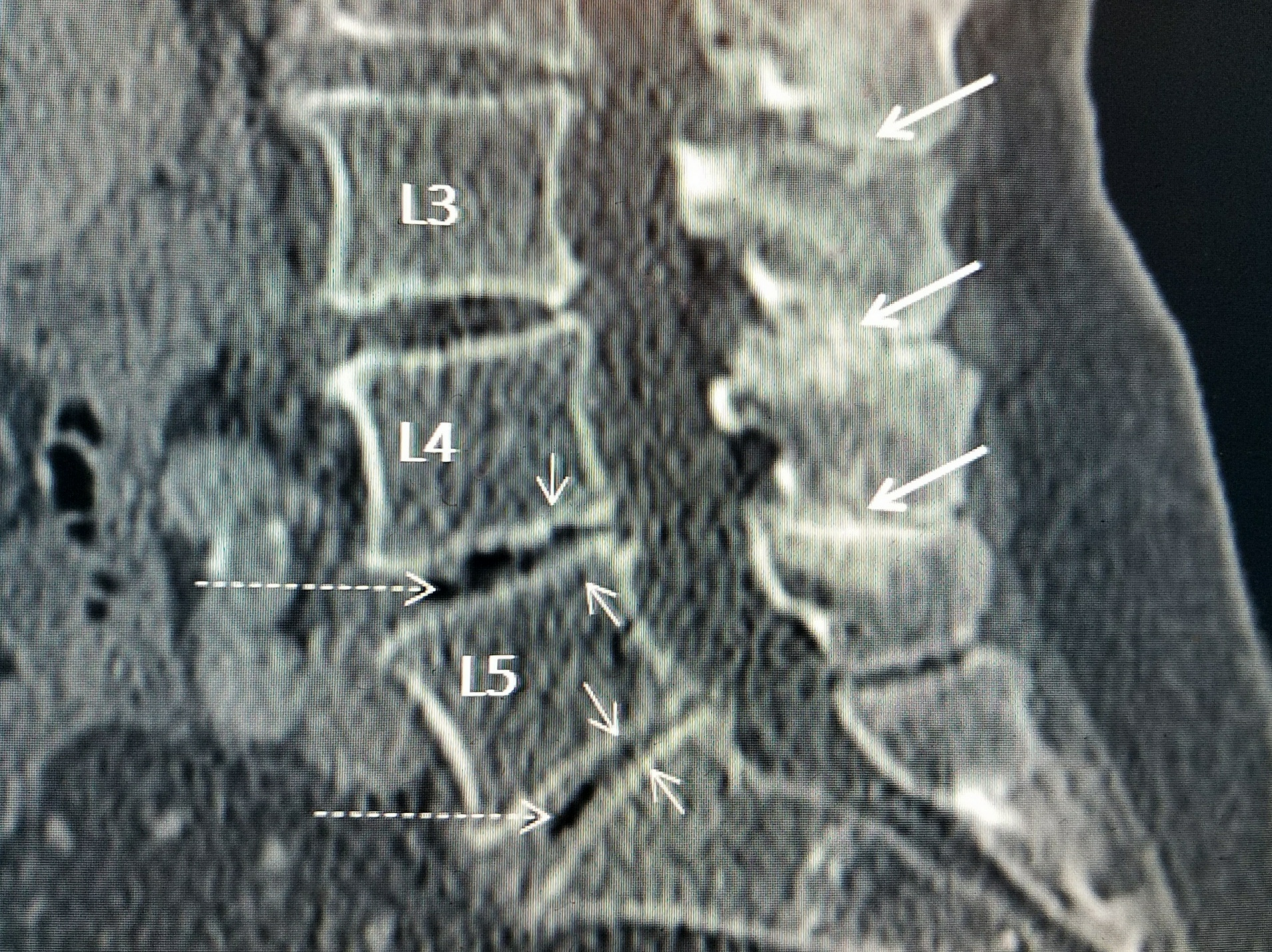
Sagittal computerized tomography (CT) scan showing 'kissing' spinous processes Sagittal lumbar CT shows the close approximation and common hyperostosis seen with the spinous processes of Baastrup's disease (thick white arrows). Also shown is associated disc space narrowing at multiple levels especially L4-5 and L5-S1 (thin white arrows) and 'vacuum' phenomenon characteristic of advanced disc degeneration (dashed white arrows) with disc necrosis and intradiscal clefts at L4-5 greater than at L5-S1.
